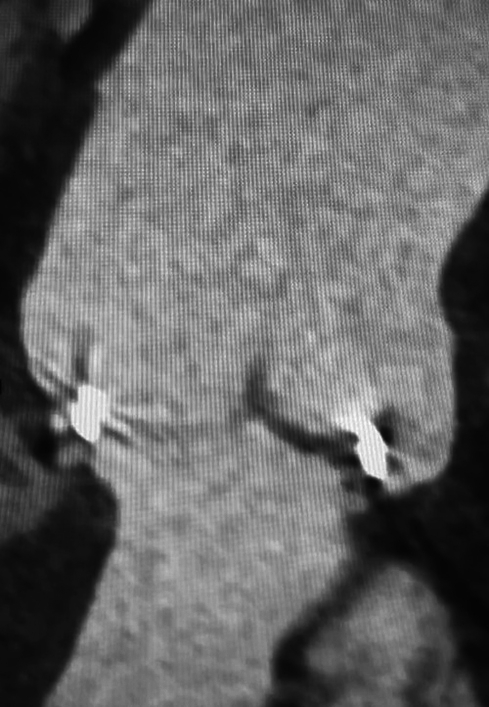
HALT visualized through CT-scan imaging on an INSPIRIS bioprosthesis at the 3-month mark.
